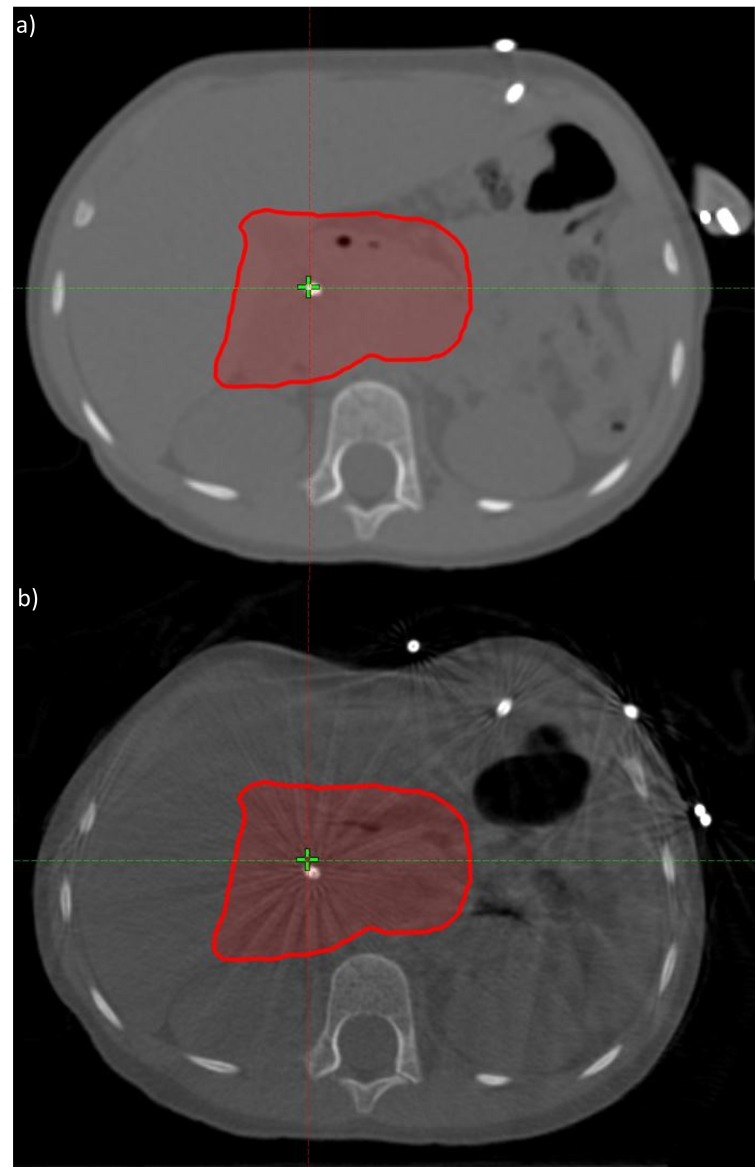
Impact of weight loss on patient body contour or circumference. a) planning CT and b) CBCT evaluation CT: computed tomography, CBCT: cone-beam computed tomography
